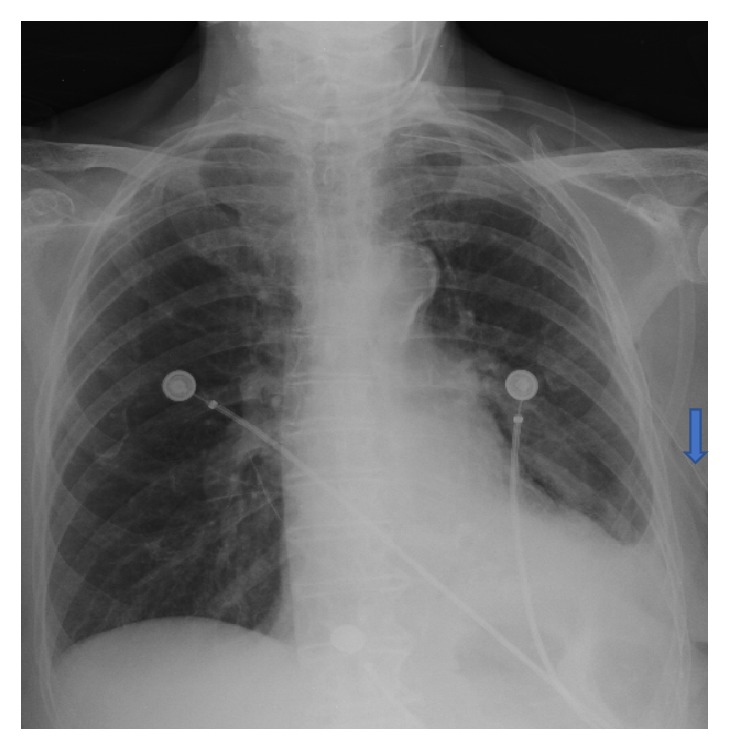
Postthoracostomy portable chest X-ray showing reexpanded left lung with residual atelectasis and or consolidation. The blue arrow points to the chest tube near the insertion point.
